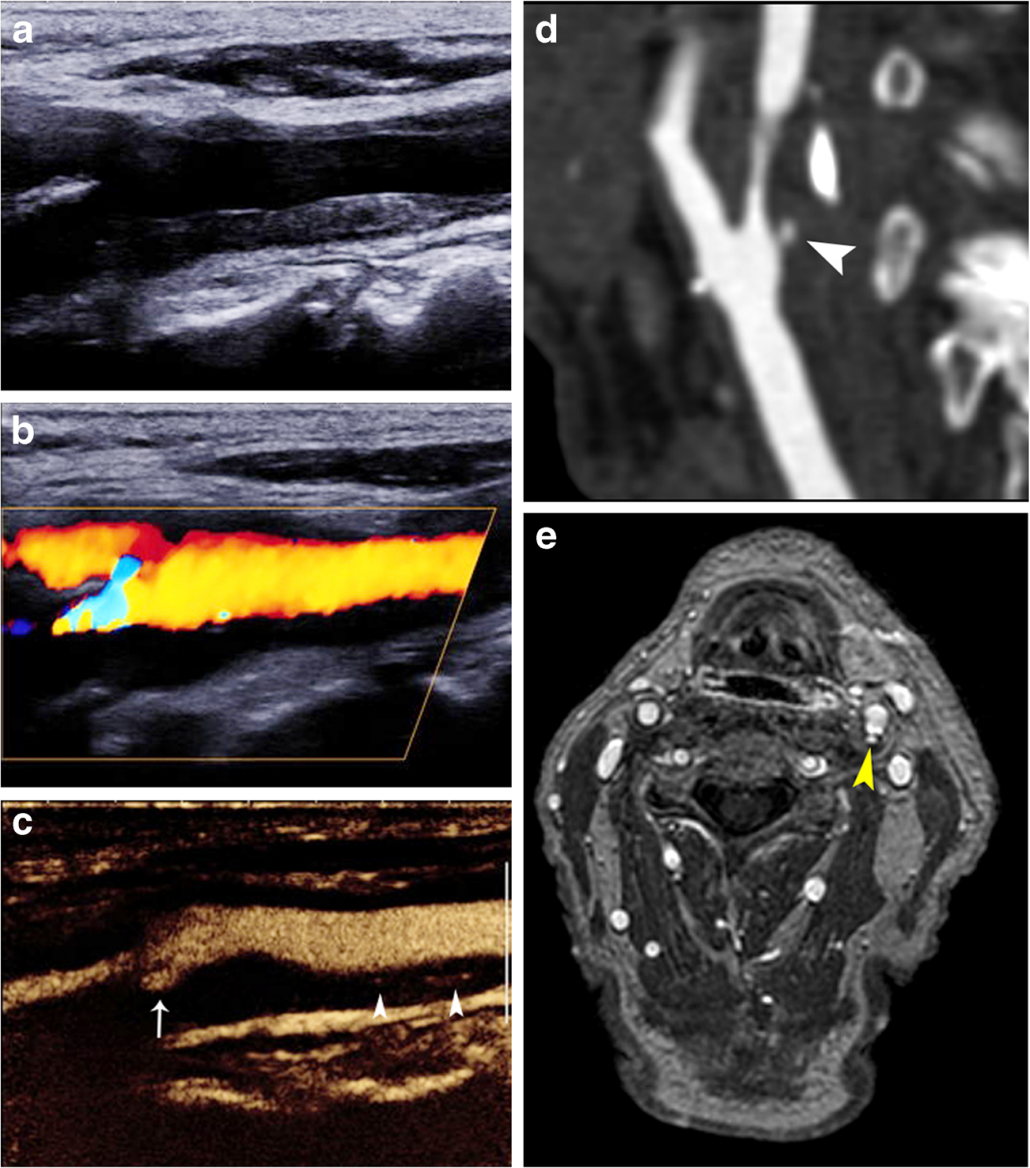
Imaging findings in a symptomatic patient with ulcerated carotid plaque. B-mode image (a) showing a smooth atherosclerotic plaque, appearing predominantly hypoechoic. Colour Doppler image (b) confirming the plaque’s smooth surface and showing severe luminal stenosis of the internal carotid artery. Note that the flow is not adequately visualised in the internal carotid artery as parts of the lumen contain no flow signals. CEUS (c) improved visualisation of blood flow in the whole field of view and provided detailed delineation of the plaque surface. Note the presence of a small superficial ulceration (*arrow*) and intraplaque neovessels (*arrowheads*). MDCTA (d) confirming the presence of a small ulceration (*arrowhead*) in an otherwise smooth plaque causing stenosis of the internal carotid artery. Axial contrast-enhanced T1-weighted MRI image (e) of the same patient performed 1 week later for follow-up of stroke confirmed the presence of ulceration (*arrowhead*)
